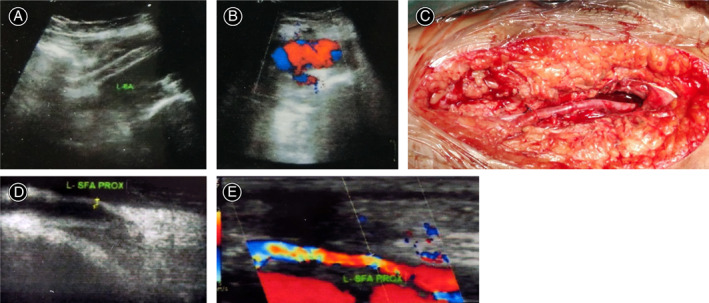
A 17‐year‐old patient with a dilated and pulsatile mass in the ilioinguinal fossa. (A, B) Duplex sonography reveals the PA. (C) The common femoral artery is repaired with autogenous saphenous vein graft interposition and sac excision. (D, E) Duplex sonography demonstrates a patent common femoral artery 4 months after operation
